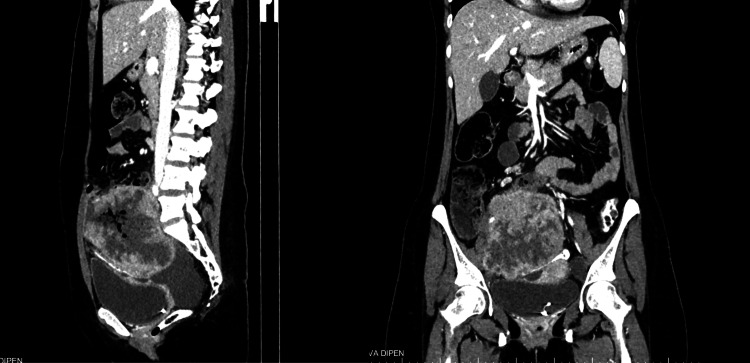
Sagittal and coronal contrast-enhanced CT scan showing a large well-defined heterogeneously enhancing necrotic right adnexal mass.
